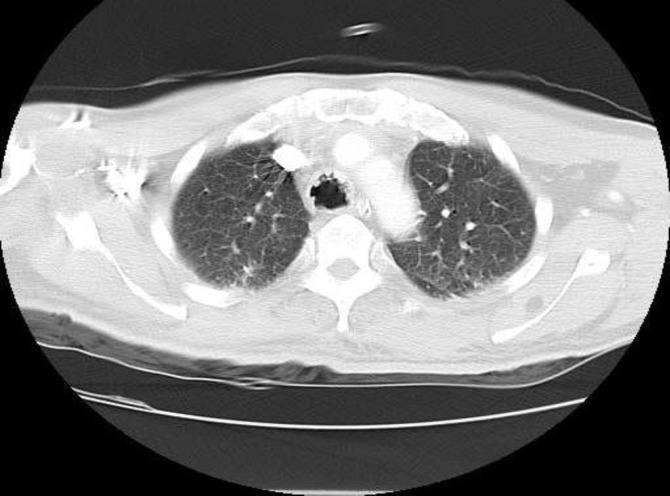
Axial contrast-enhanced CT image of trachea on lung windows showing nodular thickening of the tracheal wall
